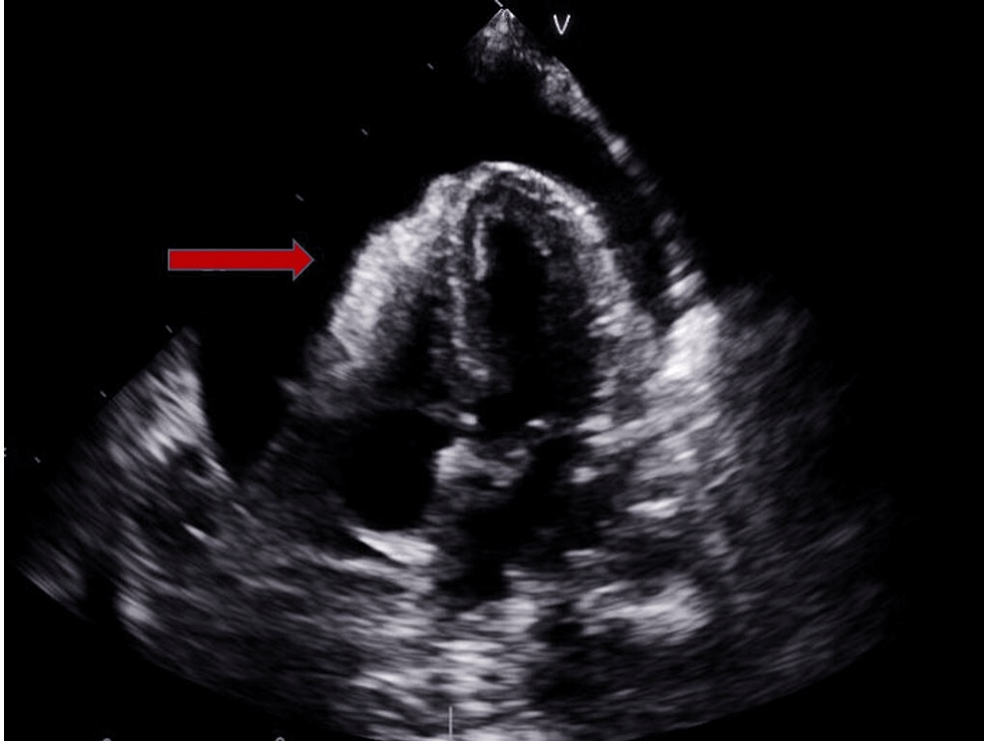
TTE showing a large pericardial effusion (red arrow) TTE - transthoracic echocardiogram
